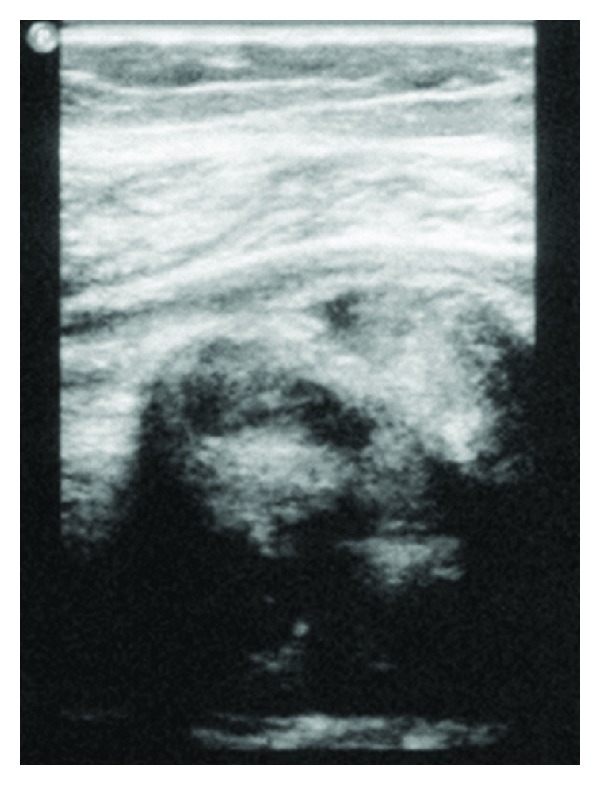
Ultrasound image of right lower leg showing a large heterogenous mass.
